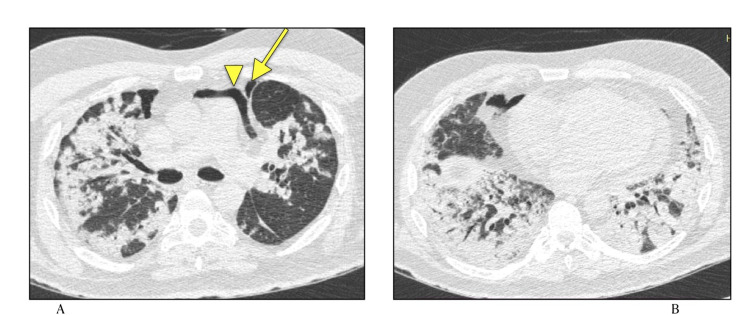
HRCT Chest (lung window) axial sections A) Pneumomediastinum (arrowhead) and pneumothorax (arrow) B) Areas of consolidation in bilateral upper lobes with few centrilobular nodules
